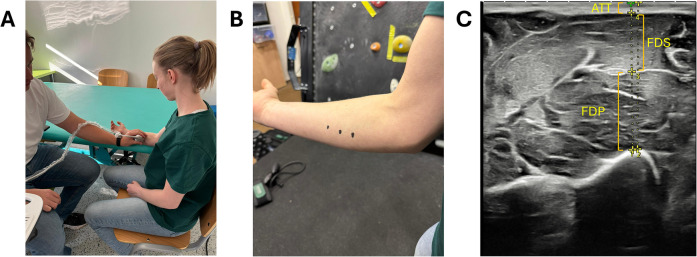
(A) The participant's position during the ultrasound measurements. (B) The marks with 20 mm intervals along the line connecting the medial epicondyle of the humerus and the lunate bone. The middle (reference) mark was placed at 33% of the distance, measured from the medial epicondyle. (C) An example of an ultrasound image and the measured tissue thickness. ATT, adipose tissue thickness; FDS, flexor digitorum superficialis; FDP, flexor digitorum profundus.
